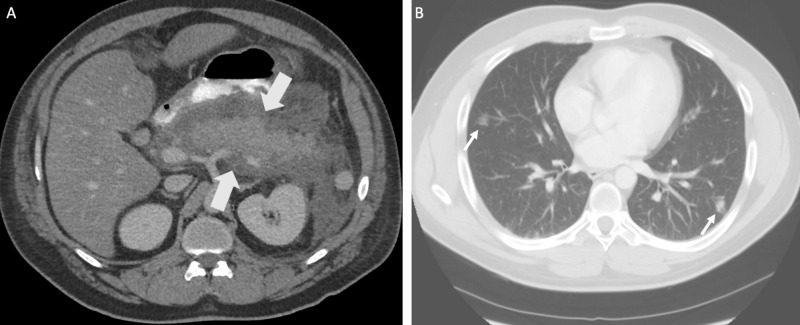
CT showing acute pancreatitis Axial (A) and (B) CT of the abdomen and pelvis with IV contrast of a 45-year-old man with abdominal pain. There is enlargement and edema of the pancreas with surrounding fluid and stranding (arrows), compatible with acute edematous pancreatitis. Ill-defined ground-glass opacities are also seen at lung bases (thin arrows in B) CT: computed tomography; IV: intravenous
